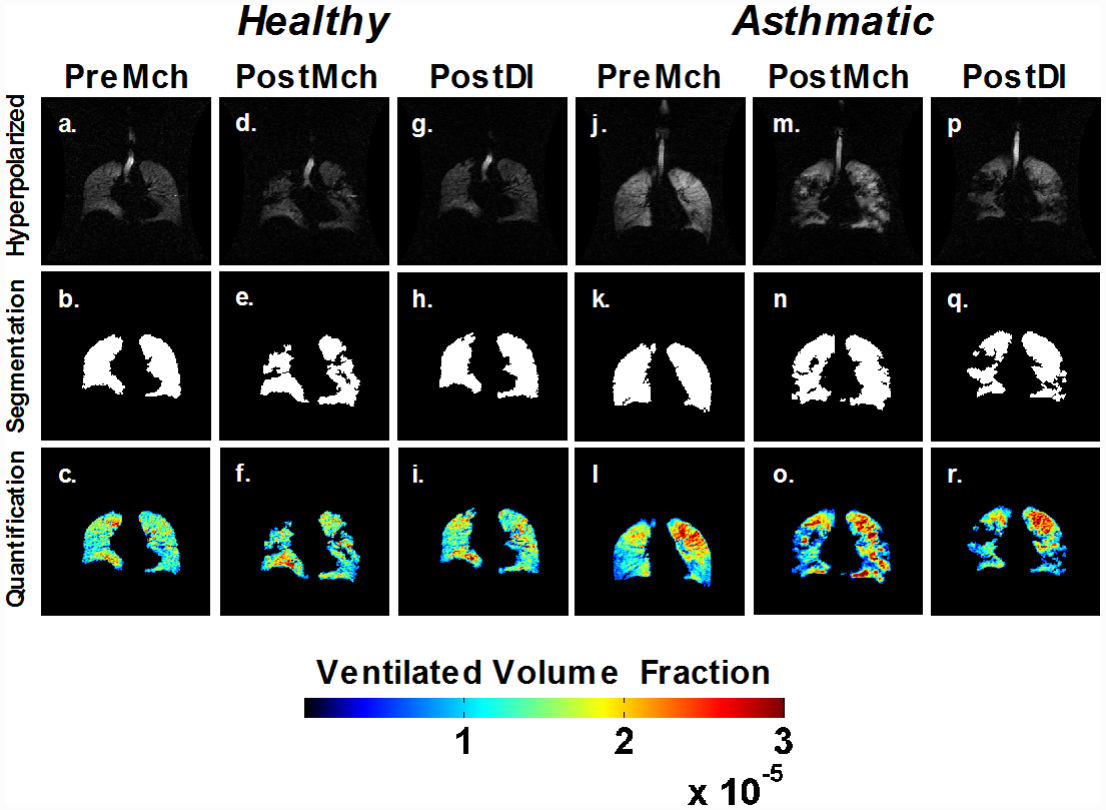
Single slice ventilation images for a healthy and asthmatic subject for PreMch, PostMch, and PostDI. HP ^3^He-MRI images (top row) are first segmented to evaluate for ventilated regions (middle row) and quantified to determine a mapped-out VVF (bottom row). Note the increased pockets of elevated ventilation in both healthy and asthmatics following a Mch-challenge and the lack of recovery for the asthmatic after a series of DIs.
